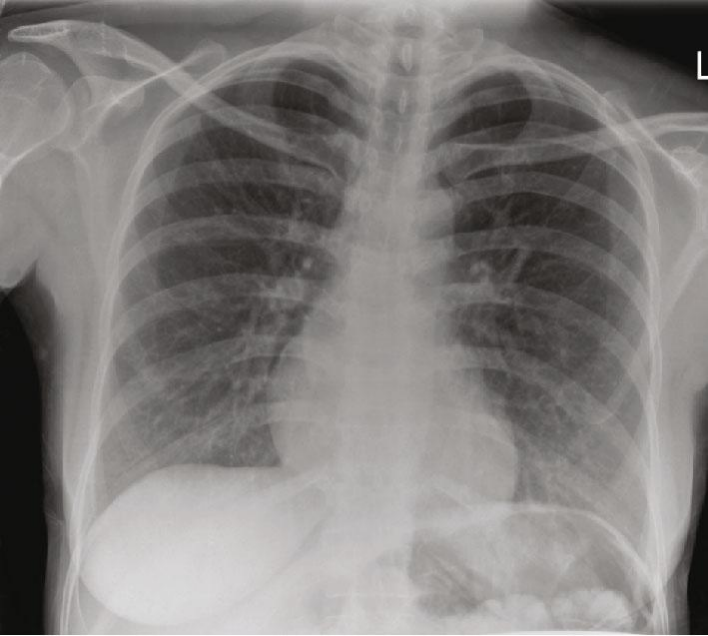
Chest X-ray showing mild bronchial thickening and minimal linear scarring in the left lung base but no definitive focal consolidation, Ghon's focus, or lymphadenopathy.
